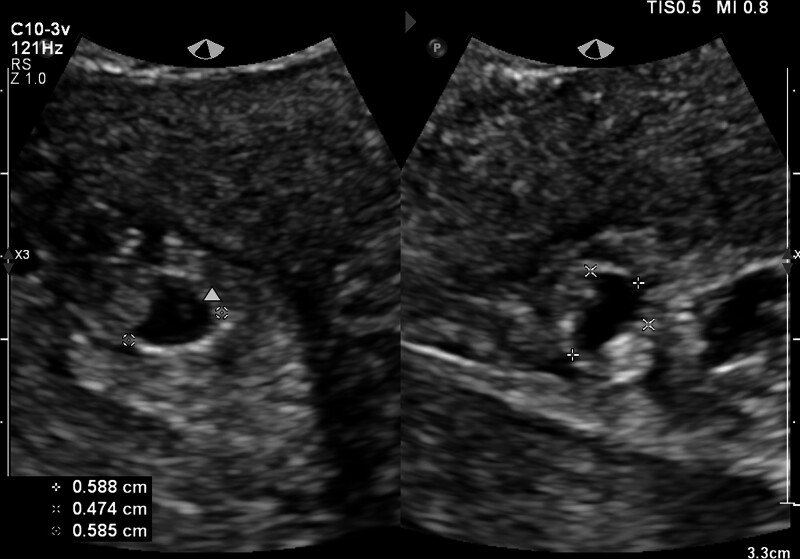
Transvaginal ultrasonography reexamination revealed a gestational sac echo near the cesarean section incision in the lower uterine segment, about 0.588 × 0.474 × 0.585 cm (*) in size, with a yolk sac inside. Arrow: yolk sac.
